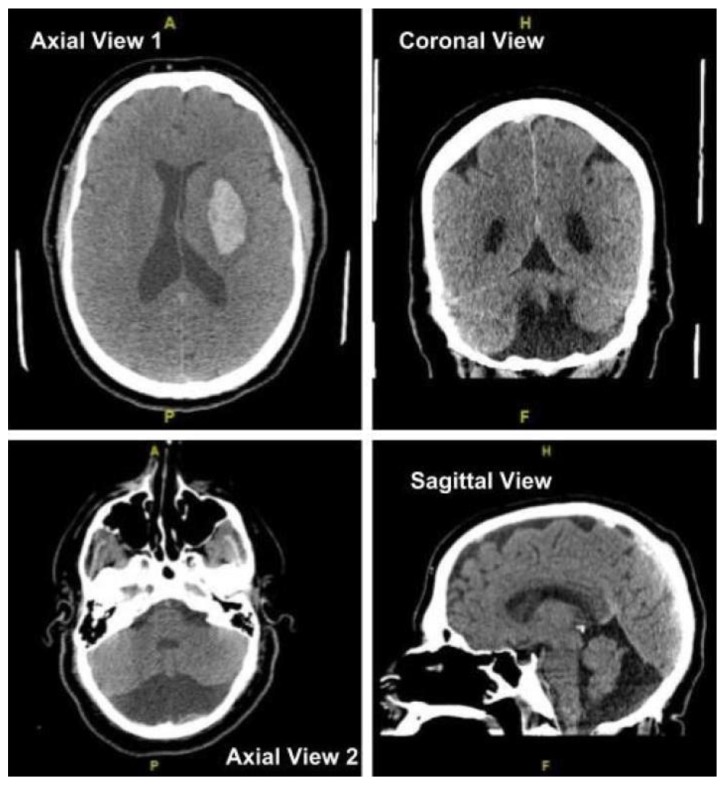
CT studies obtained upon arrival revealing left basal ganglia hemorrhage in axial view 1 and absence of the cerebellum consistent with DWM in coronal view, axial view 2 and sagittal view.
